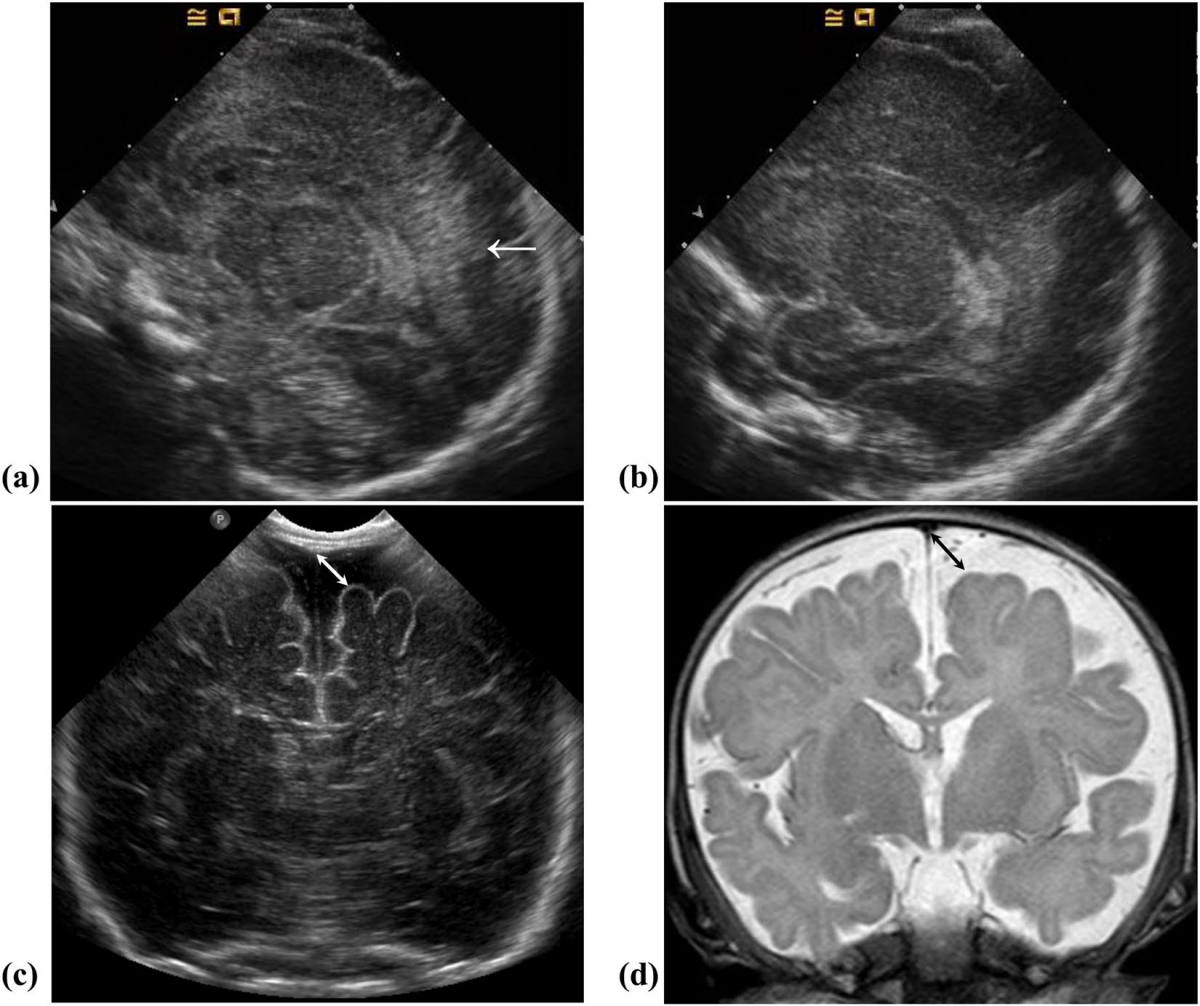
(a–d) Serial brain images of a preterm infant enrolled in our study. The infant was born at a gestational age of 29 + 5 weeks, with a birthweight of 1306 g. The initial cranial ultrasound done on the 4^th^ day of life reveals inhomogeneously increased periventricular echogenicity (arrow) to the same degree as choroid plexus (a). In the subsequent cranial ultrasound done at the 20^th^ day of life, the periventricular increased echogenicity is regressed without cystic formation (b). Enlarged subarachnoid space is first noticed on the follow-up cranial ultrasound done at 45^th^ day of life (arrow), at a corrected age of 36 + 0 weeks (sinocortical width = 8.3 mm) (c). On the brain magnetic resonance image (coronal T2-weighted image, TR/TE 5482/100) done at term-equivalent age, the enlarged subarachnoid space (arrow) is also noticed (d). The infant was screened positive in the gross motor function field at the neurodevelopmental assessment done at the follow-up visit.
